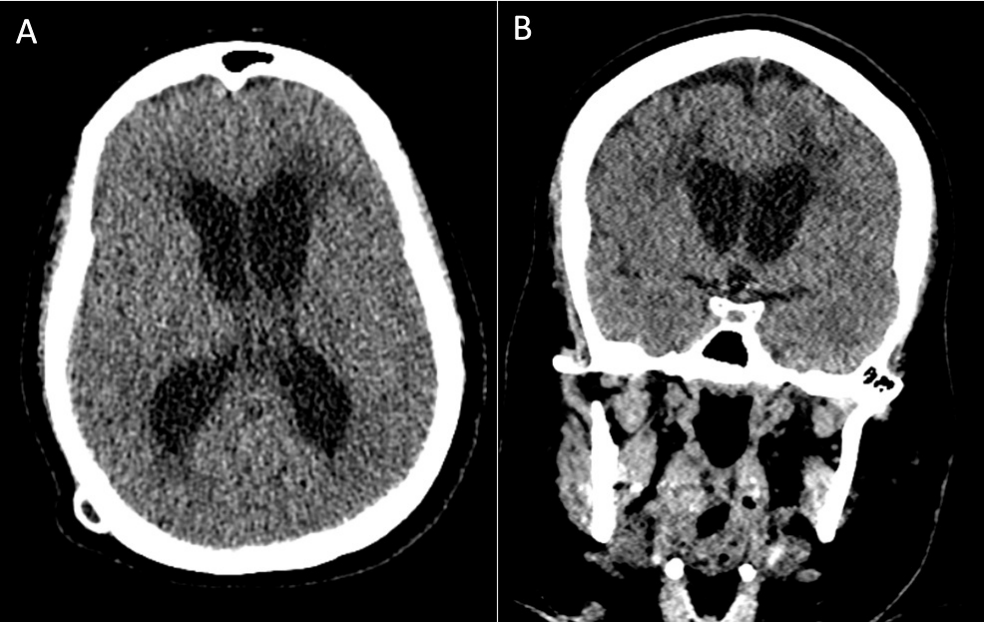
Preoperative CT imaging (A) axial and (B) coronal preoperative computed tomography (CT) scans
